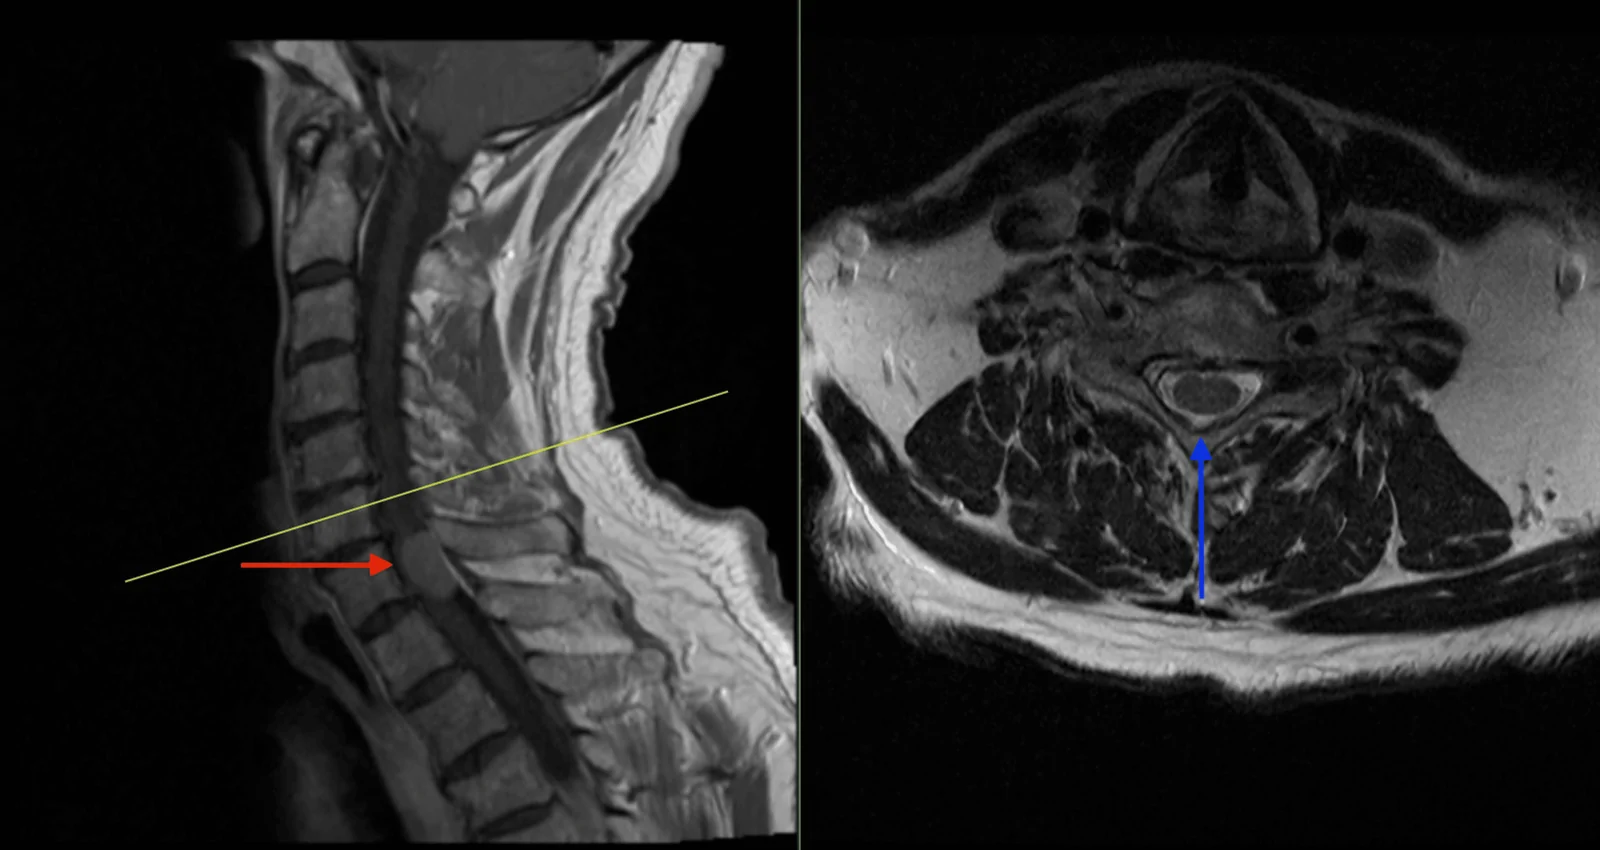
T1 with contrast spinal MRI without meningioma in axial view Sagittal (left) and axial (right) T1-weighted cervical spine MRI with contrast demonstrating normal soft-tissue and spinal canal anatomy without evidence of a meningioma. The sagittal slice plane is indicated by the yellow line.
